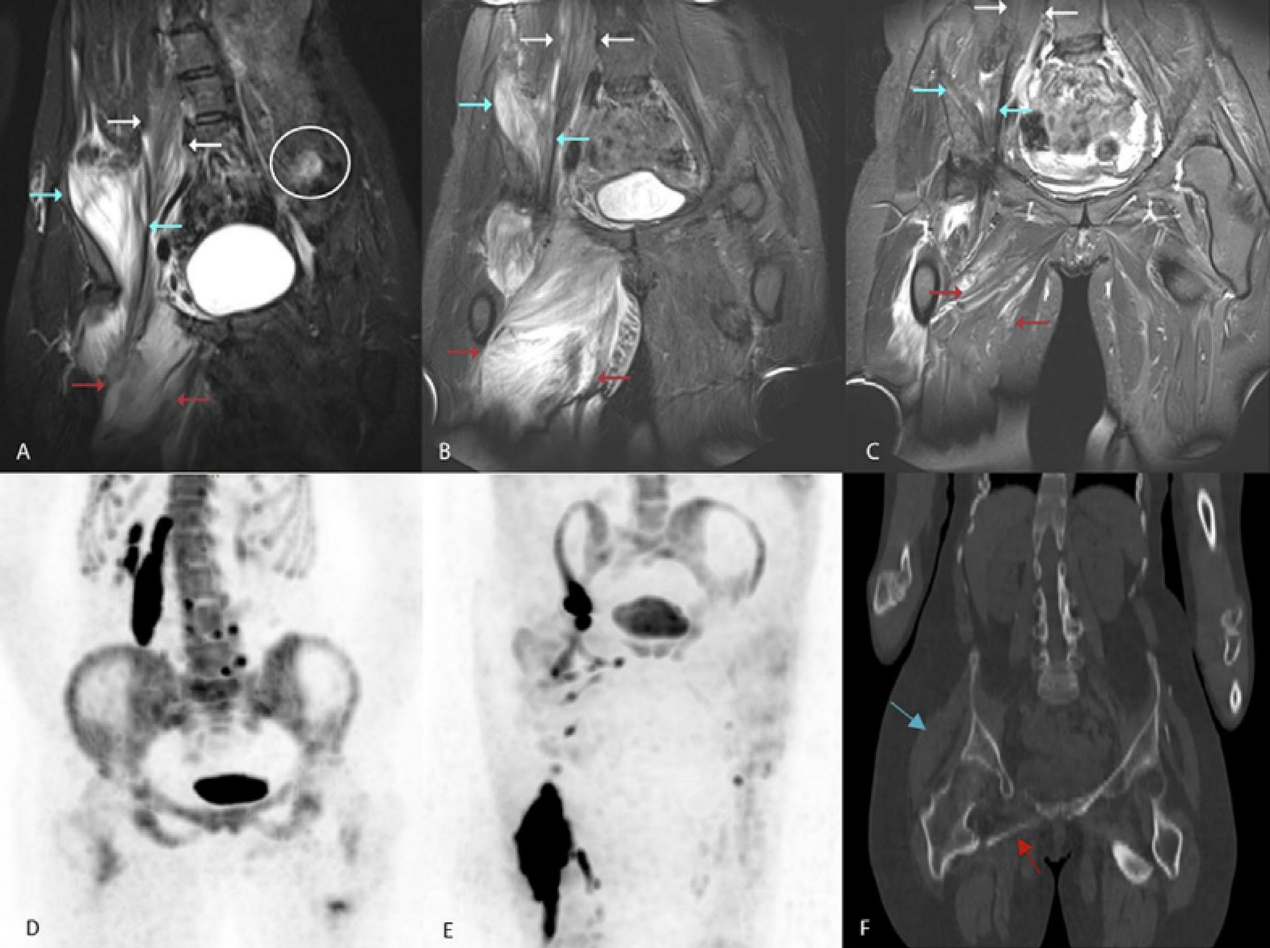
Consecutive MRI scans and [^18^F]NaF PET scans of a patient with several flare‐ups. Coronal MRI T2‐weighted short‐TI inversion recovery (STIR) images are shown of a patient with multiple flare‐ups. Starting in the loin (*A* + *D*), later also the groin (*B*) and upper leg (*C* + *E*). Time in months. *A* and *D*; T = 0. Clinically, a flare‐up in the pelvic area with pain and swelling of the entire right loin. MRI (*A*) showed moderate and severe edema of the musculus psoas dextra (white arrows) and the musculus iliacus dextra (blue arrows), respectively. Also, the musculi adductors (red arrows) showed moderate edema, even though no clinical signs were noted. The MRI showed also an area of nonspecific mild edema (white circle). [^18^F]NaF PET showed increased high uptake of tracer in the psoas muscle, mild uptake in the mm. adductors and no uptake in the iliopsoas muscle. *B*; T = 1. Edema at both the musculus psoas and musculus iliacus diminished, to mild and moderate edema, respectively. Edema intensity at the musculi adductors increased to severe, the patient now reported flare‐up symptoms at the groin too. The mild edema seen in plane A resolved; no calcifications were noted. *C* and *E*; T = 11. Edema at the psoas muscle, and the iliacus and adductor muscles is completely resolved, but new edema formed in the quadriceps muscle (*C*). High [^18^F]NaF uptake in the quadriceps (*E*). *F*; T = 21. Low‐dose whole‐body CT showed heterotopic ossification (HO) in the psoas muscle, HO at the site of the adductor muscles (red arrow), and in the quadriceps muscle. No HO formed in the iliopsoas (blue arrow).
